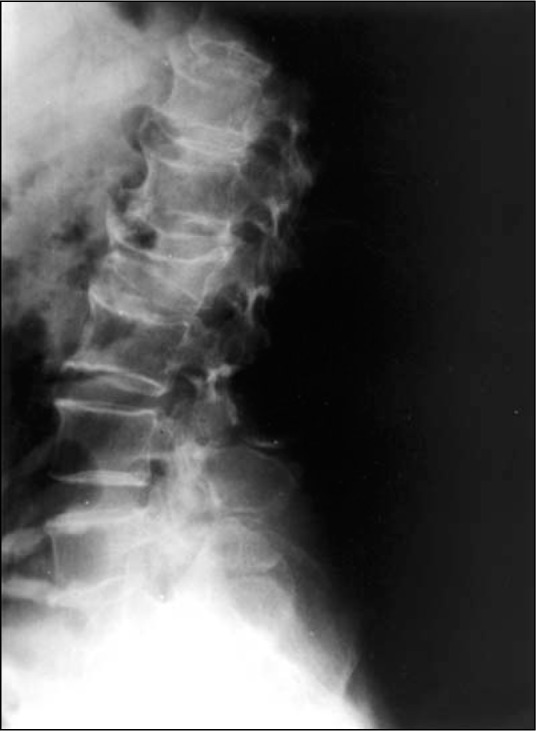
Lumbar spine x-ray showing lumbar fracture in L_2_ and multiple and large osteophytes.
